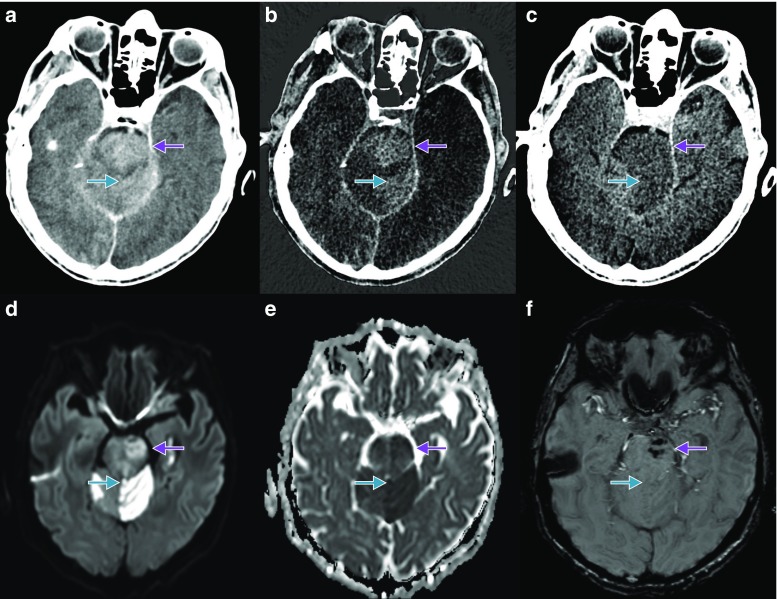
a–f Example of a patient with a left cerebellar haemispheric infarction (*blue arrow*) and a left pontine infarction (*violet arrow*), which became haemorrhagic 2 days after the IAR. The infarctions are hyperdense on BW series (Figure 1a), hyperdense on IM series (Figure 1b), hypodense on VNC series (Figure 1c), hyperintense on diffusion weighted imaging (DWI B1000, Figure 1d), hypointense on the apparent diffusion coefficient map (ADC map, Figure 1e), hypointense on susceptibility weighted imaging (pontine, haemorrhage), or isointense (cerebellar, no haemorrhage; Figure 1f) in the follow-up imaging
